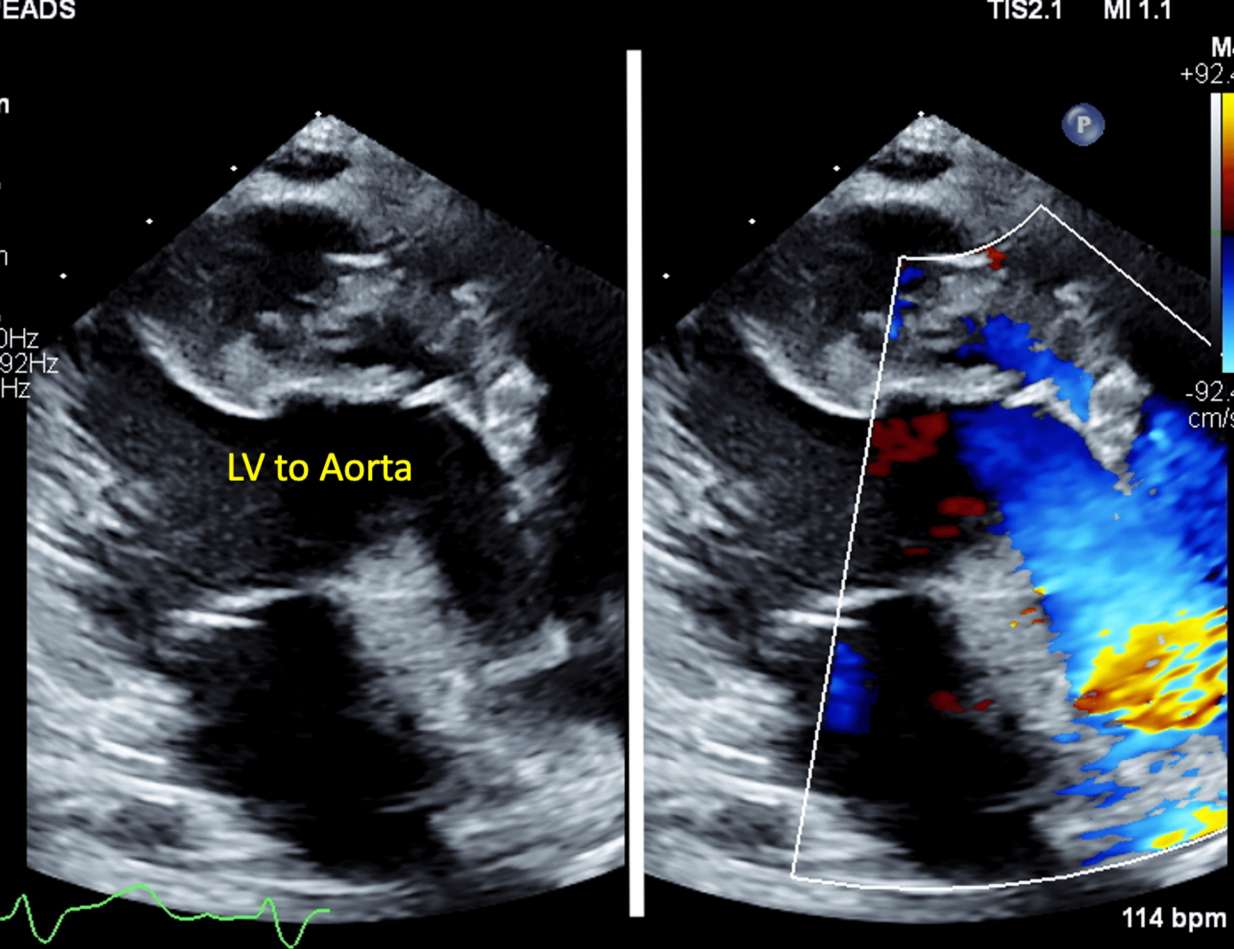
Postoperative echocardiogram (parasternal long axis view) showing the patent left ventricular outflow tract LV, left ventricle
